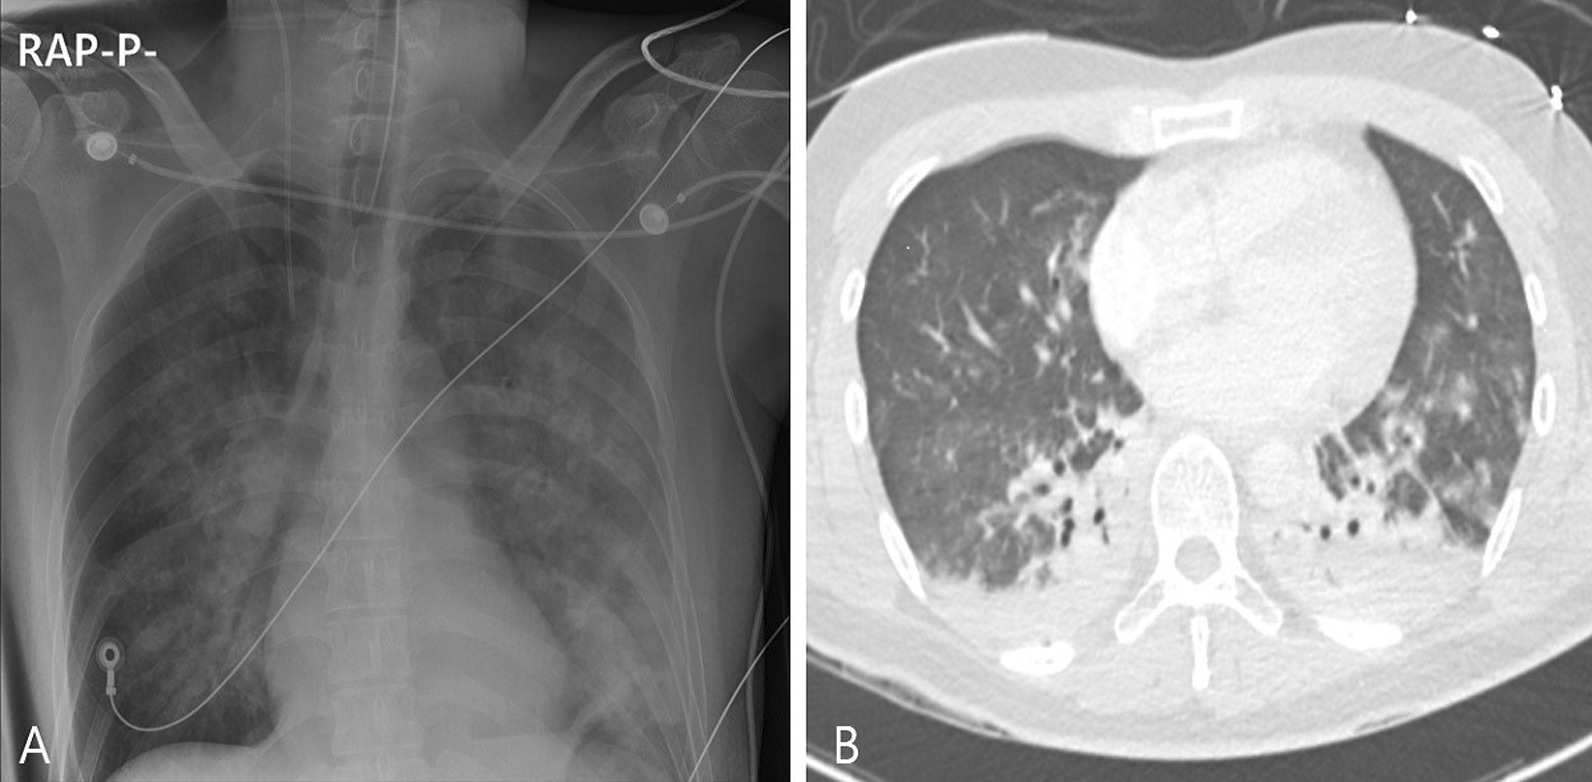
Chest imaging findings. A Bilateral infiltrates on chest X-ray. B Anterior–posterior density gradient by consolidation in bilateral dependent area with background diffuse ground-glass opacities
